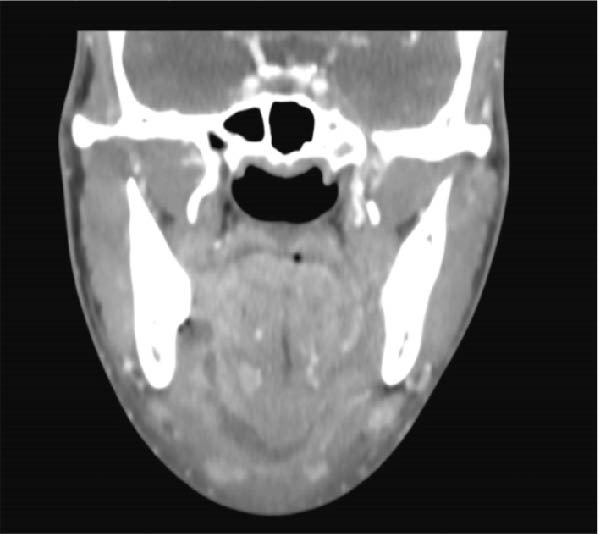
Contrast‐enhanced coronal CT of Case 1 demonstrating involvement of the right pterygomandibular, submandibular, and submental spaces.
